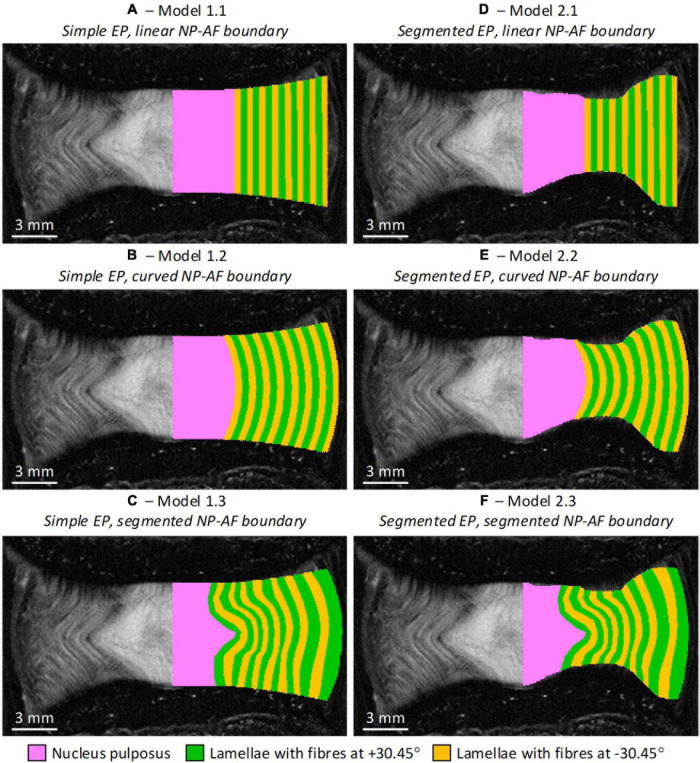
Initial geometry of the six axisymmetric FE models overlaid on the MRI slice from which the geometries were obtained. Note the axis of symmetry in the models is along the mid-sagittal plane of the IVD. (A) Model 1.1—simple endplates, linear NP-AF boundary, (B) Model 1.2—simple endplates, curved NP-AF boundary, (C) Model 1.3—simple endplates, segmented NP-AF boundary, (D) Model 2.1—segmented endplates, linear NP-AF boundary, (E) Model 2.2—segmented endplates, curved NP-AF boundary, (F) Model 2.3—segmented endplates, segmented NP-AF boundary. Note the fibre bundle angles are relative to the transverse plane.
